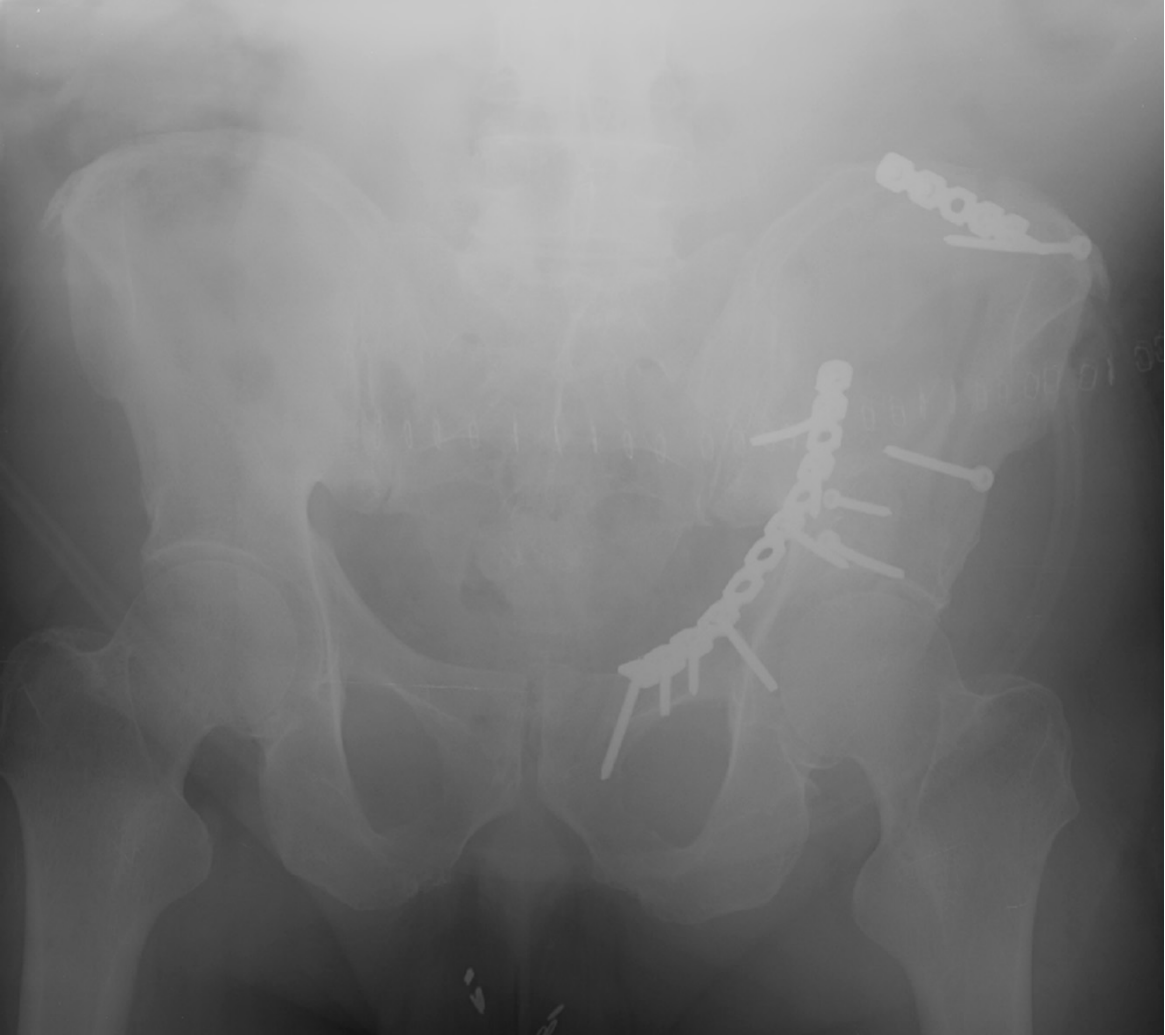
Immediate postoperative AP and oblique pelvic radiographs demonstrating near anatomic reduction status post ORIF.
